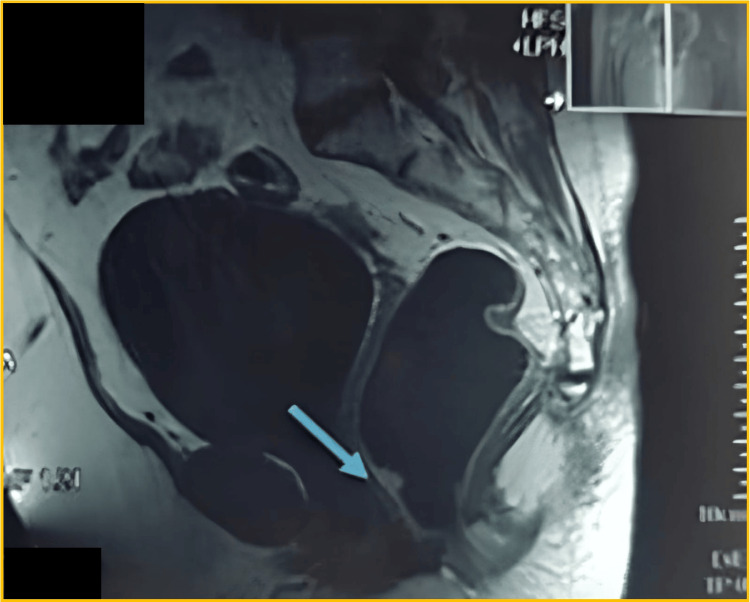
Pelvic MRI T2-weighted sagittal view of the pelvic MRI showing a hyperintense, pseudonodular lesion (blue arrow) arising from the anterior wall of the anal canal, approximately 2 cm from the anal verge. The lesion measures 1.5 cm in diameter and is consistent with a vascular malformation.
